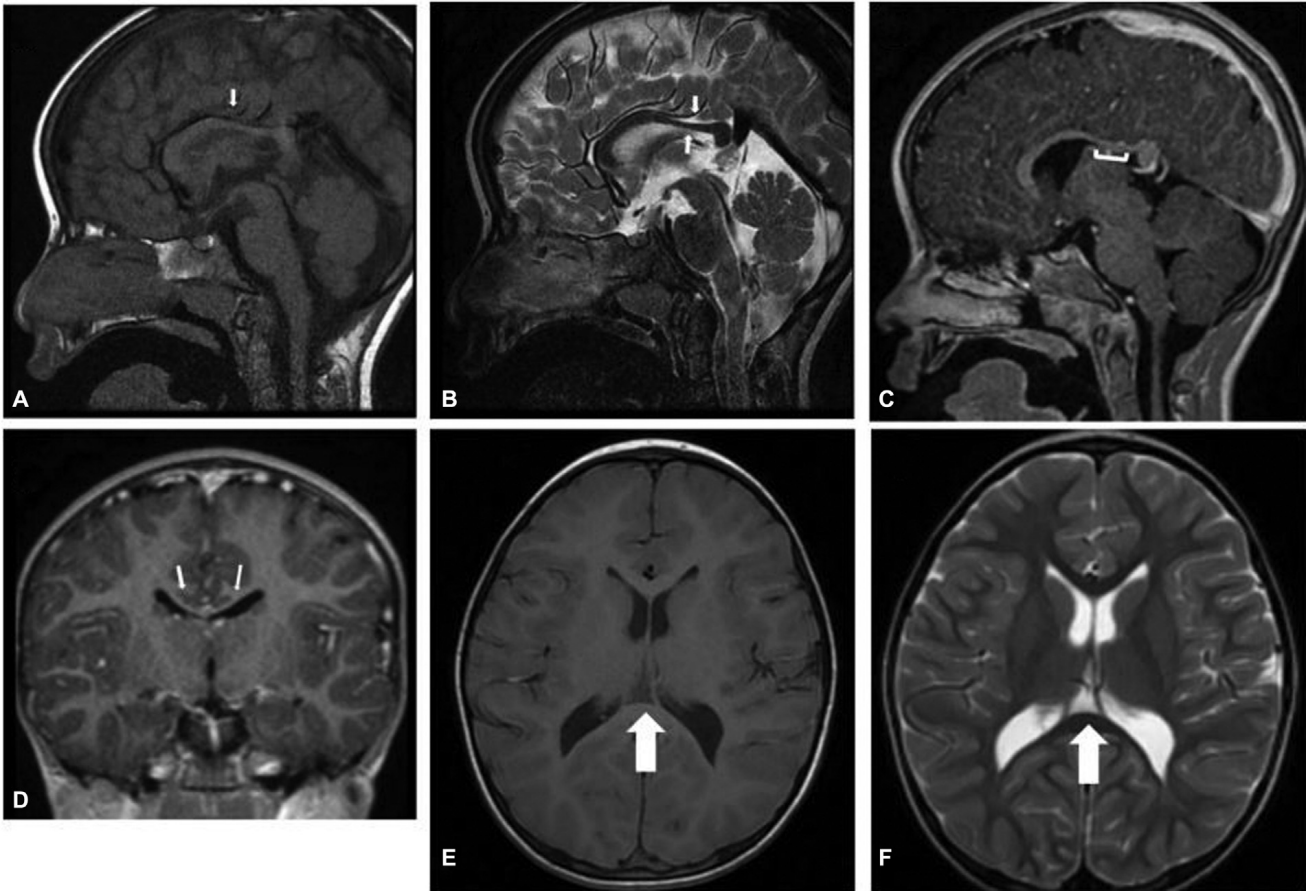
MRI of a 3.5 years-old boy with 12q21 deletion and dysmorphism of the corpus callosum
